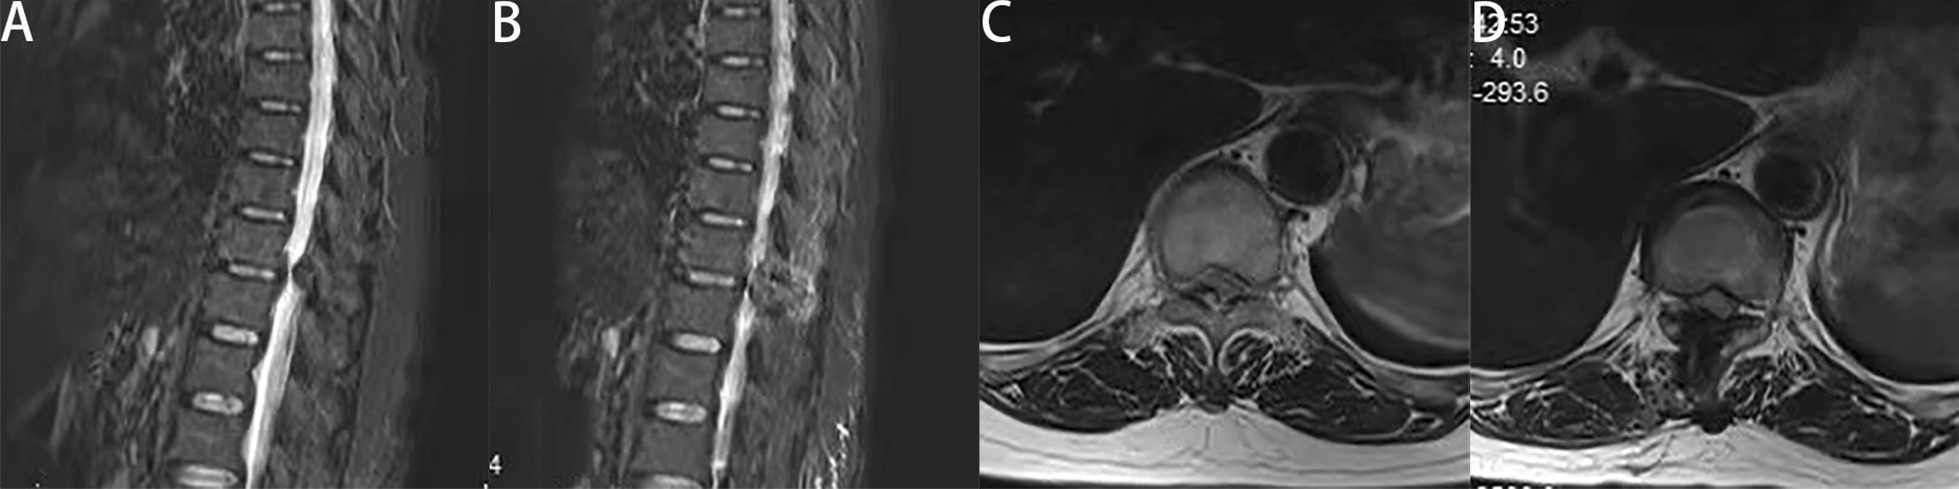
Satisfactory decompression was assessed by comparing the preoperative (A, C) and postoperative (B, D) MRI
